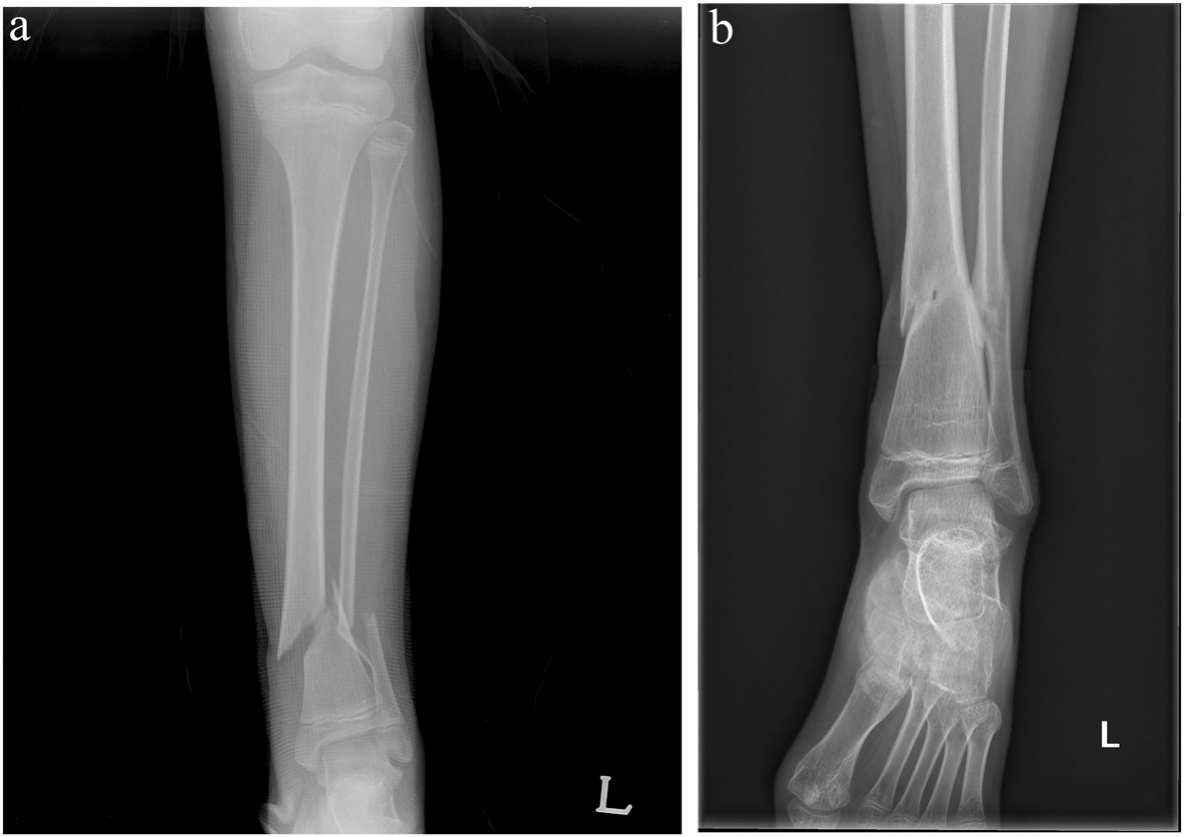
Radiograph of a ten-year-old girl who sustained a left distal tibiofibular fracture. (a) Anteroposterior radiograph at trauma. (b) A radiograph after 3 years of follow-up shows tibiofibular synostosis and an ankle valgus deformity associated with fibular shortening.
